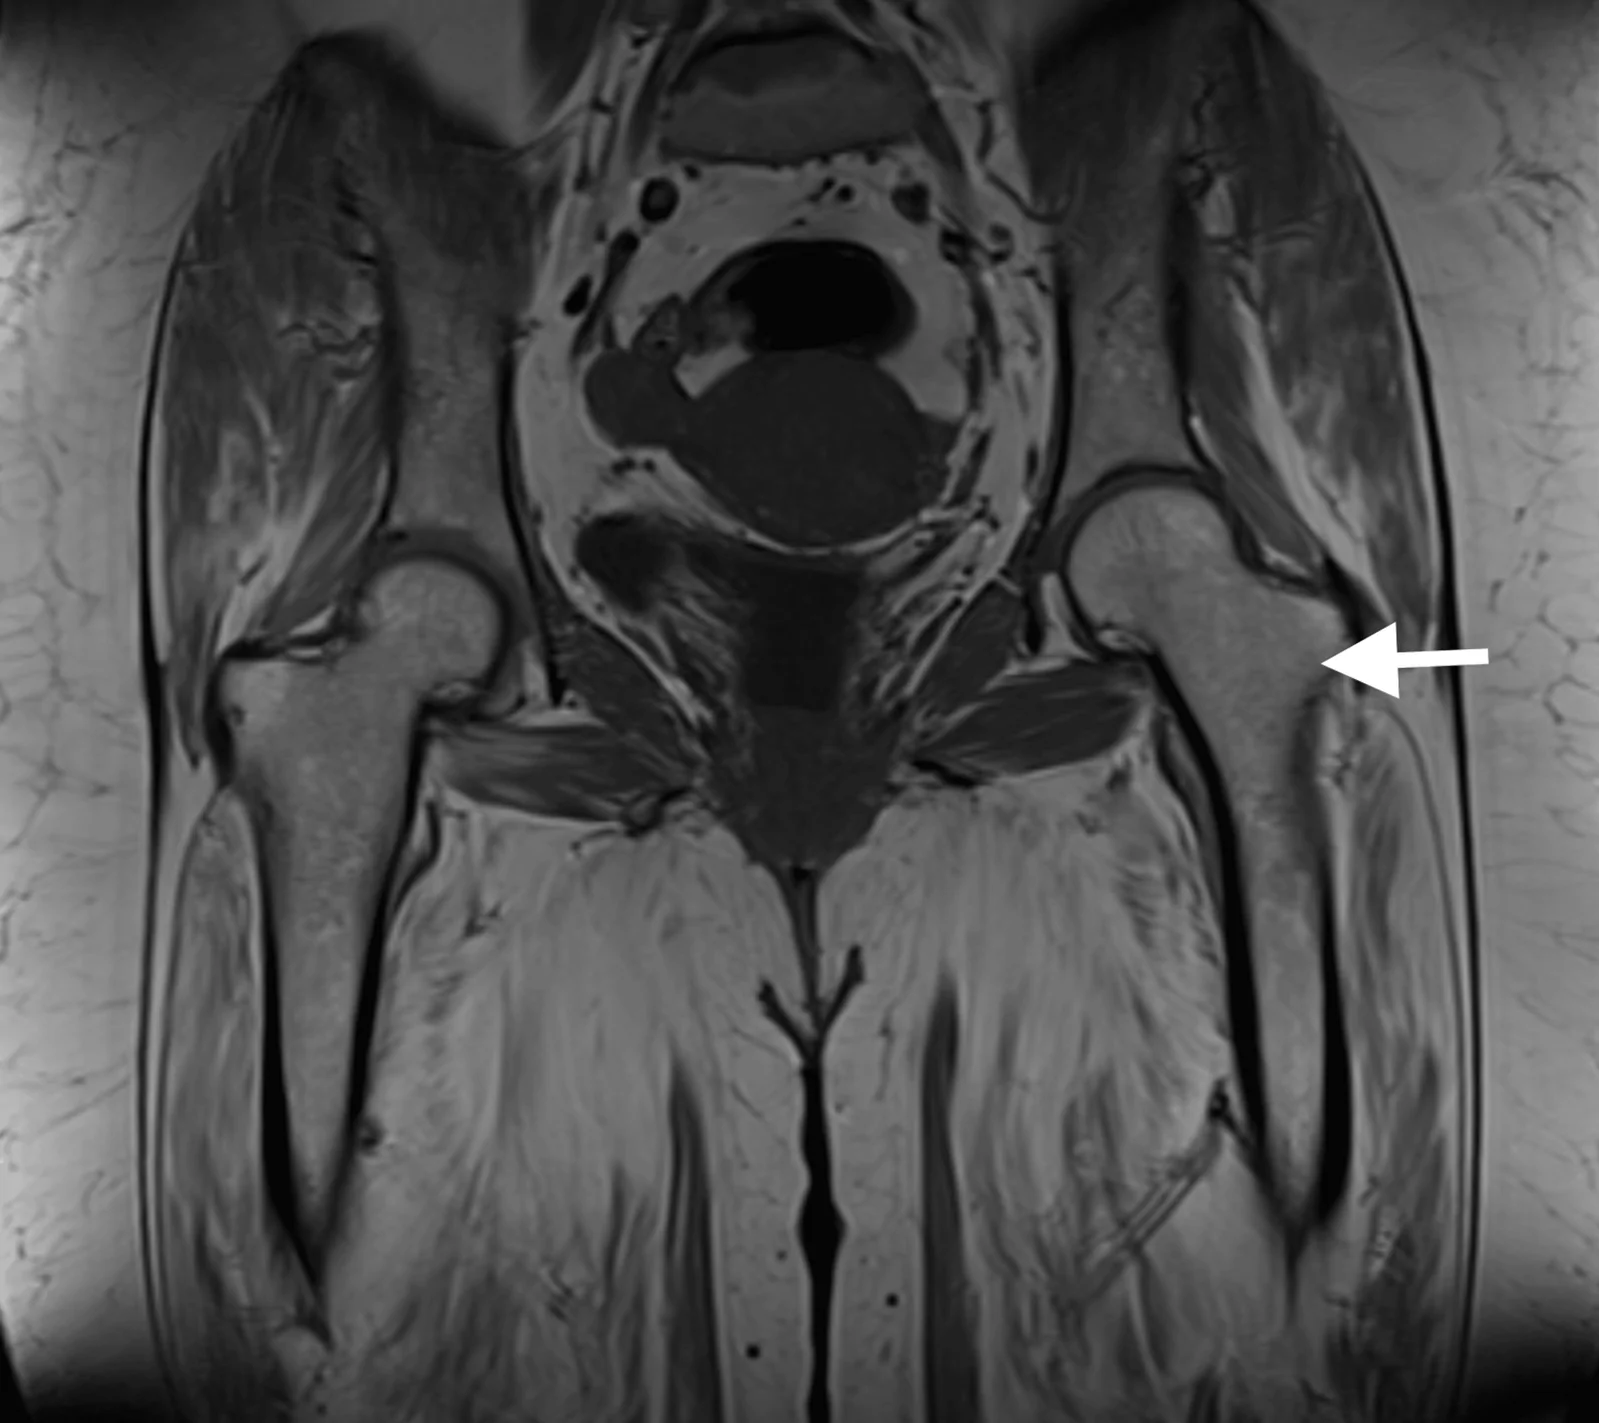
Coronal T1-weighted follow-up MRI of the pelvis showing resolution of the previously observed lesion, with no residual abscess (arrow). The follow-up MRI shows no evidence of residual inflammation or abscess at the previously affected site, as indicated by the arrow. No residual disease is identified.
